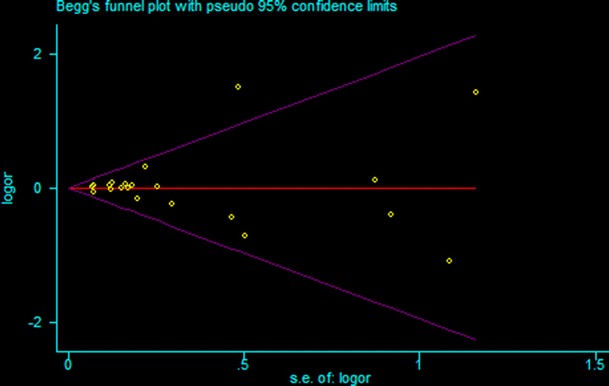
Funnel plot for publication bias of the meta-analysis of cancer risk and DNMT3B −149C/T polymorphism (CC/CT vs. TT)
